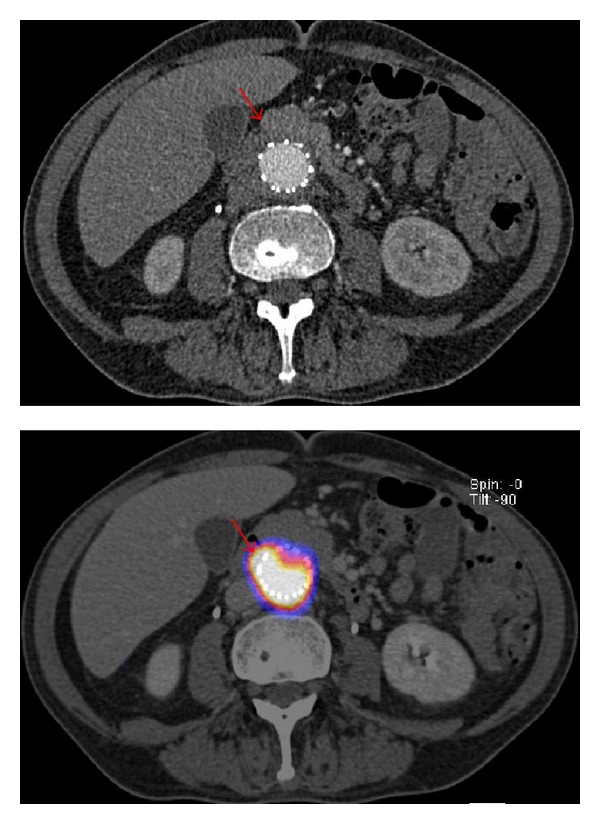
Axial view of a CT scan and fused ^18^F FDG PET/CT image of a culture proven infected vascular prosthetic graft. In this particular case, a 67-year-old male patient underwent an urgent endovascular procedure of a contained ruptured abdominal aortic aneurysm using a Cook Zenith prosthesis. After 7 months the patient was readmitted with clinical signs of a prosthetic infection. CT scan was assessed as a possible prosthesis infection. An ^18^F-FDG PET/CT scan confirmed the diagnosis by showing an increased FDG uptake at the level of the endograft. Ultimately,* Escherichia coli* were cultured from the graft.
